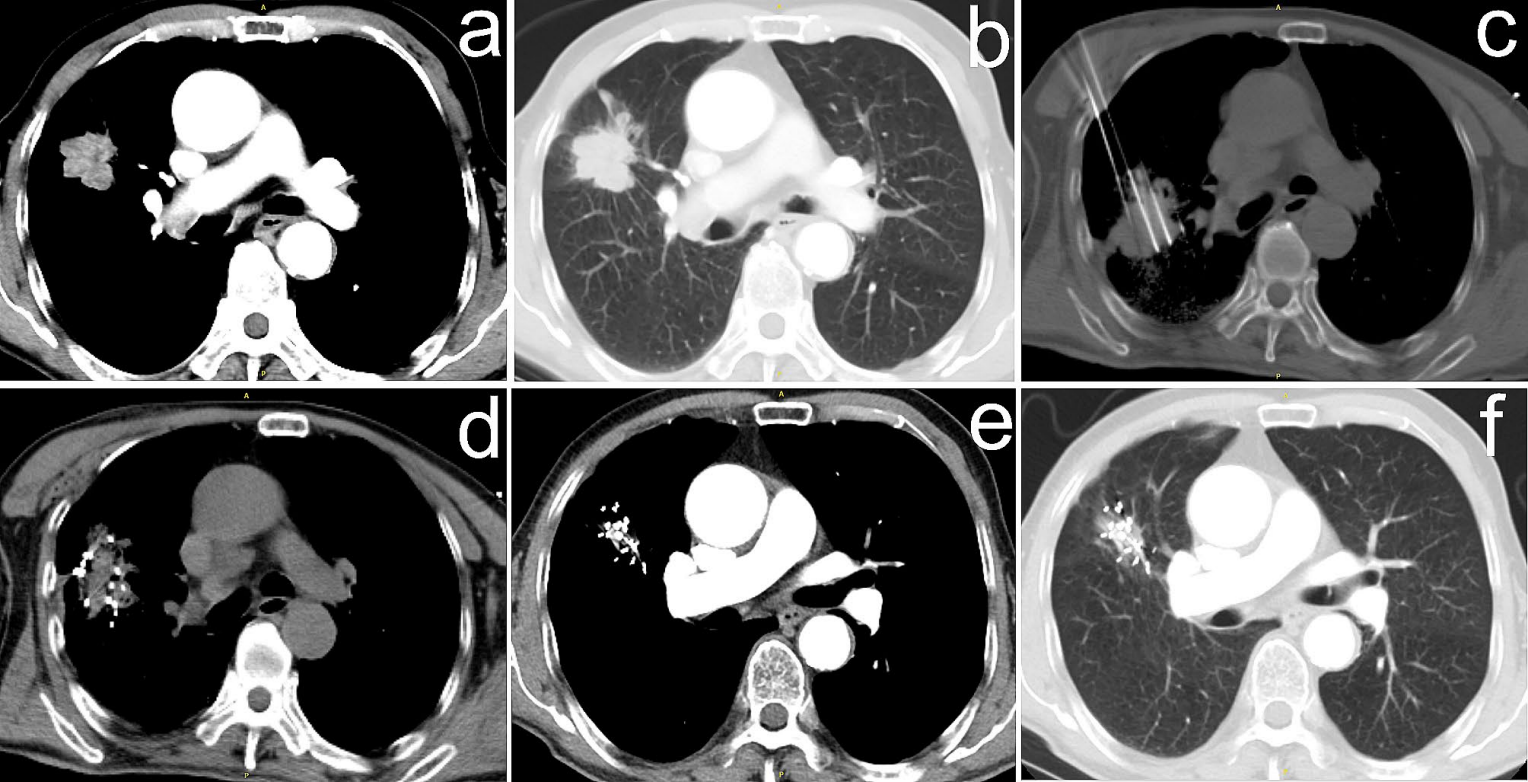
(a) Thoracic CT demonstrated a mass in the right lung inferior lobe before treatment; (b) Lung window; (c) Multiple needles were inserted into the lesion under CT guidance; (d) Iodine-125 seeds were implanted into the tumor; (e) Follow-up CT 24 months after the procedure showed complete response; (f) Lung window
